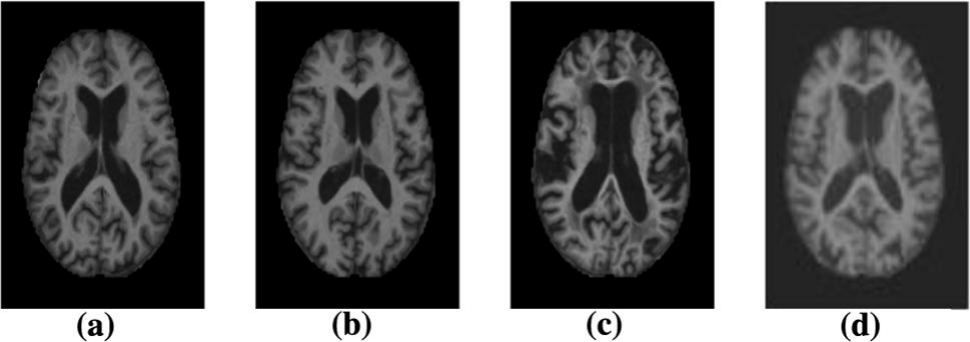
Example of different brain MRI images presenting different Alzheimer’s disease stages. a Non-demented; b Very mild dementia; c Mild dementia; d Moderate dementia
